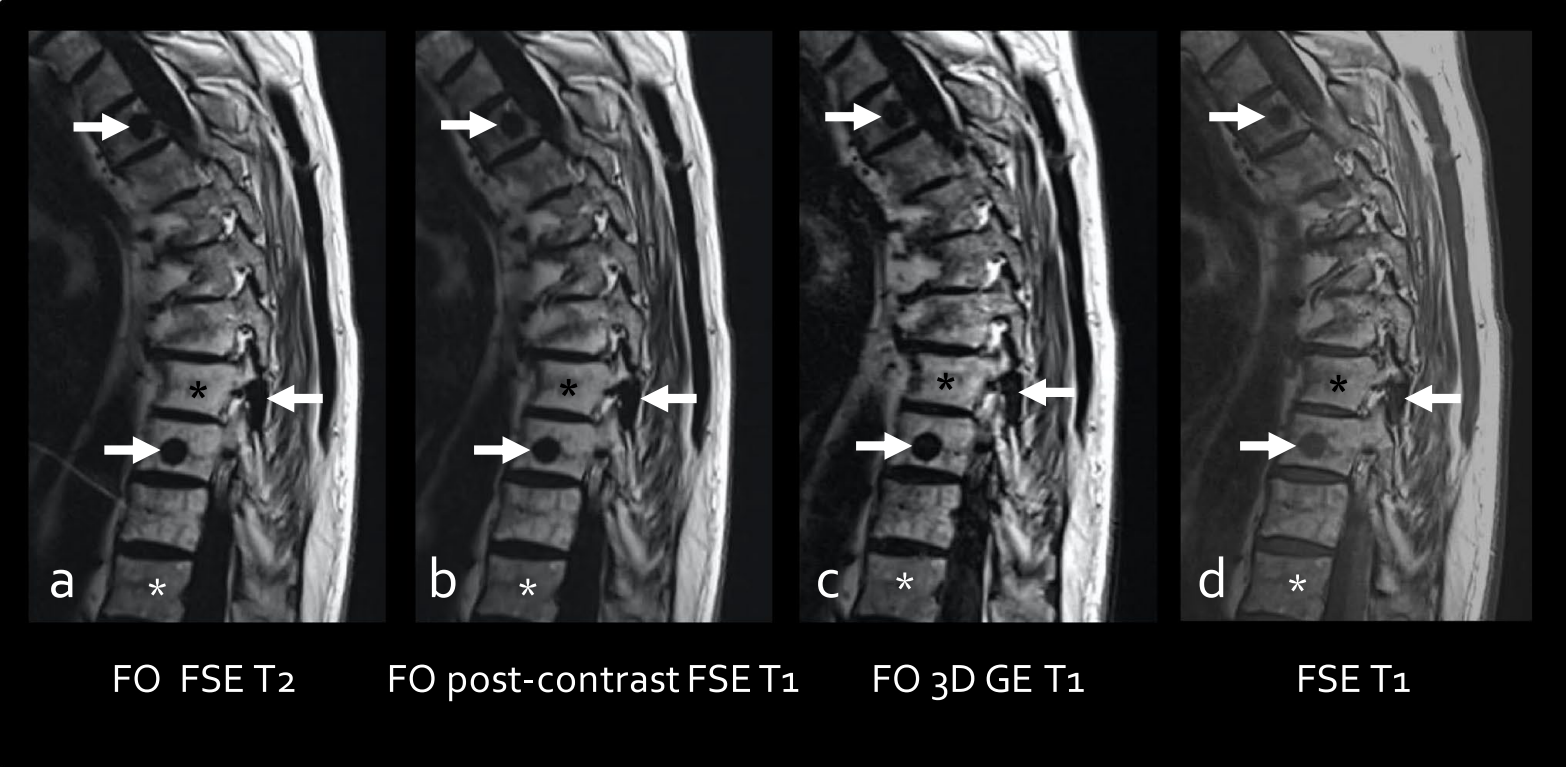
64-year-old male with a history of breast carcinoma treated with surgery, chemo- and radiotherapy. Fat only (FO) (a–c) and FSE T1-weighted (d) images show multiple metastases (arrows) presenting as signal void on FO images. FO images from a FSE T2-weighted (a), post-contrast FSE T1-weighed (b), and post-contrast 3D gradient-echo T1-weighted sequences (c) are almost superimposable. Note the higher contrast between the lesions (arrows) and surrounding tissues, as well as between fatty marrow (post-radiotherapy) (black asterisk) and red marrow (white asterisk) on FO images (a-c) compared to the FSE T1-weighted image (d). Based on the data from the literature, our spine protocols in the sagittal plane (workup of metastases, degenerative spine, spondyloarthritis) have been reduced to a single FSE/TSE fluid-sensitive Dixon sequence providing four sets of images [3, 11–14]
